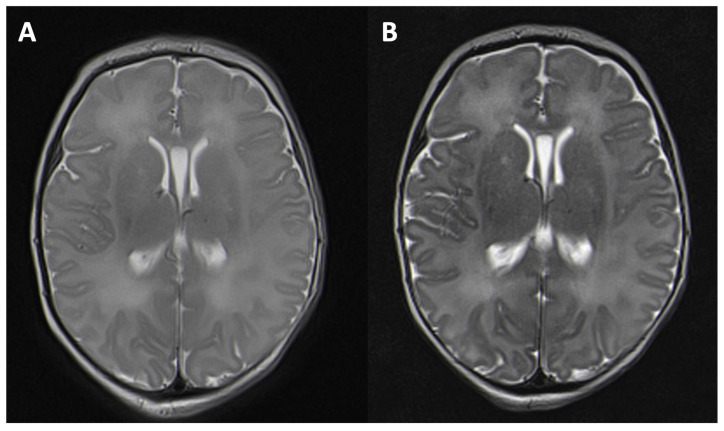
T2-weighted image in an axial orientation of a six-day-old girl after asphyxia. A higher grey–white matter contrast can be seen with a longer echo time of 203 ms, repetition time of 5500 (B) than with a medium echo time of 113 ms, repetition time of 6950 (A).
